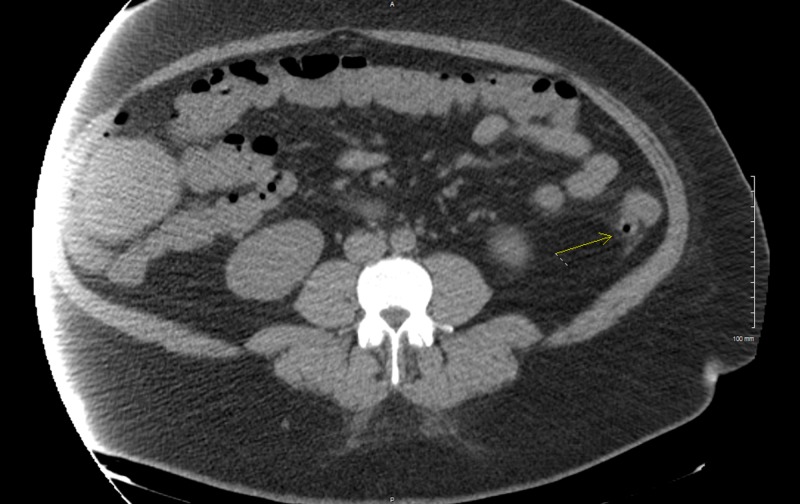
Computed tomography (CT) scan showing diverticula (yellow arrow) in the descending colon with fat stranding
